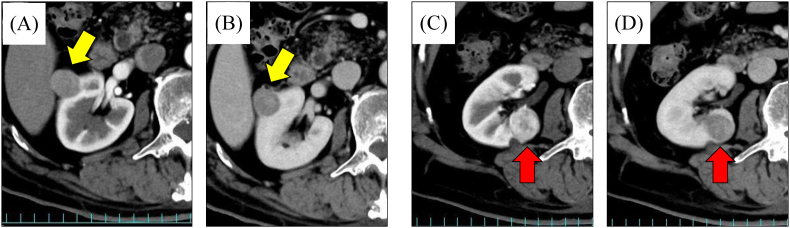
Enhanced CT showed a tumor measuring 20 × 19 mm on the ventral side of the right kidney (the yellow arrow indicated). The tumor showed no enhancement during the early (A) or the late (B) phases. Enhanced CT showed a tumor measuring 21 × 20 mm on the renal hilum of the right kidney (the red arrow indicated). The tumor showed strong contrast effect at the early phase (C), with disappearance of this effect at the late phase (D).
